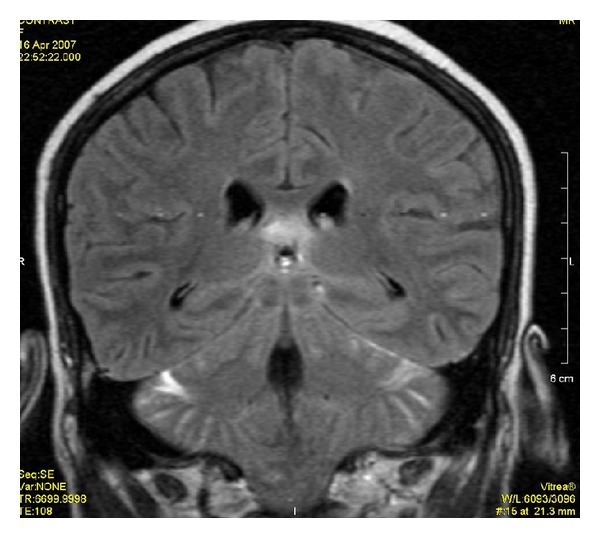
Postcontrast FLAIR image shows meningeal enhancement along cerebellar cortex and along left tentorium.
